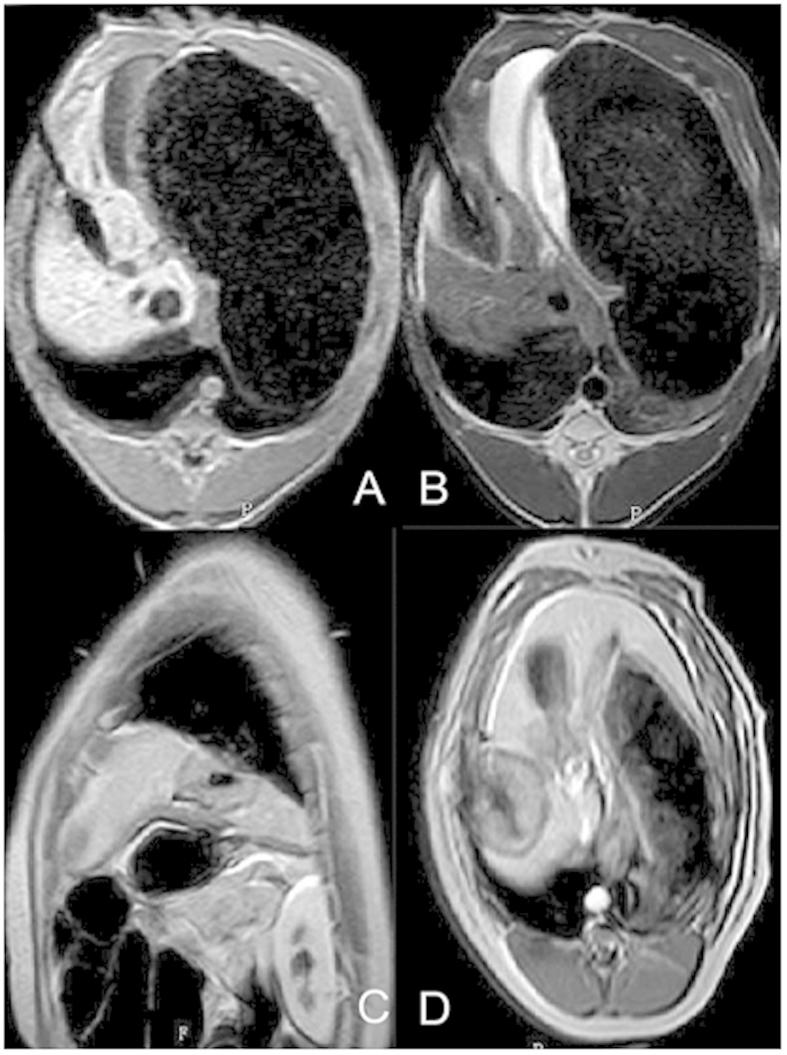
Imaging characteristics after microwave ablation. The ablated area represented a thin oblong hypointense oval around the antenna on T1W images (A) and thick oval hypointense with hyperintense surroundings on T2-weighted images (B). On contrast-enhanced T1-weighted images, the ablated area is represented by a thin line hypointense in the center, while enhancement of the ablated area was hypointense (C,D).
